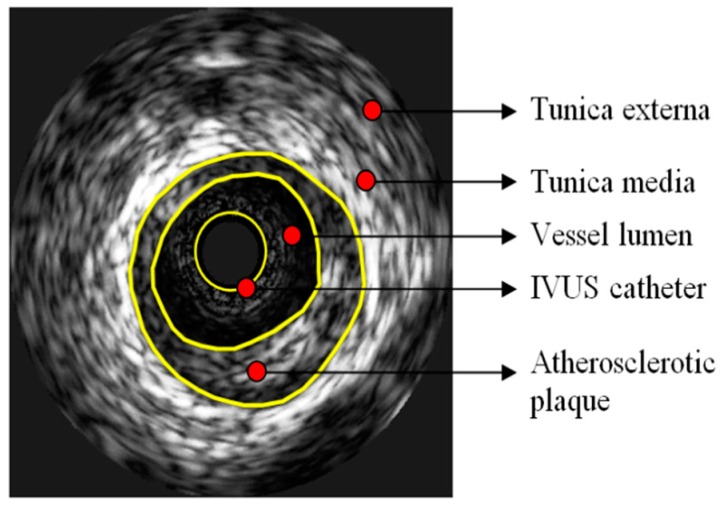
Typical conventional grayscale display of an image acquired through intravascular ultrasound imaging (IVUS). Hippokration Hospital, 1st Department of Cardiology, National and Kapodistrian University of Athens.
